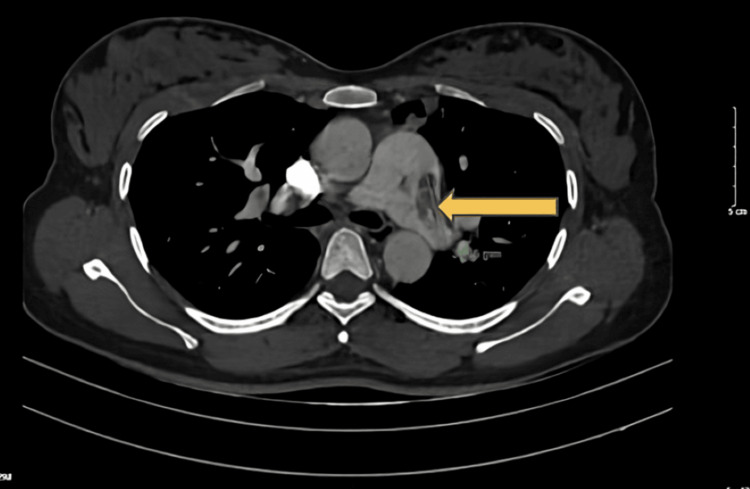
Vegetation on a plain chest CT scan
